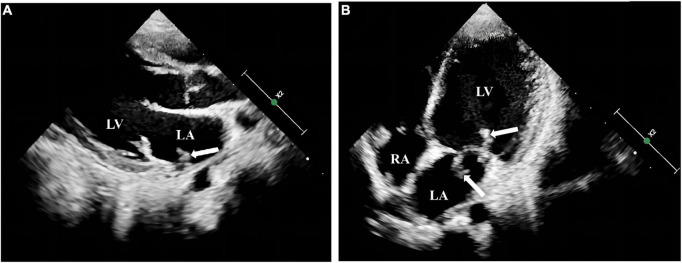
Transthoracic echocardiography examination of the heart in the pediatric patient. A striated flocculent hyperechoic attachment (A), was observed at the entrance to the left pulmonary vein in the lateral posterior wall of the left atrium, with thickened anterior and posterior mitral leaflets and a striated flocculent hyperechoic attachment with a floppy texture visible in both anterior and posterior leaflets (B). LA: left atrium; LV left ventricle; RA: right atrium.
